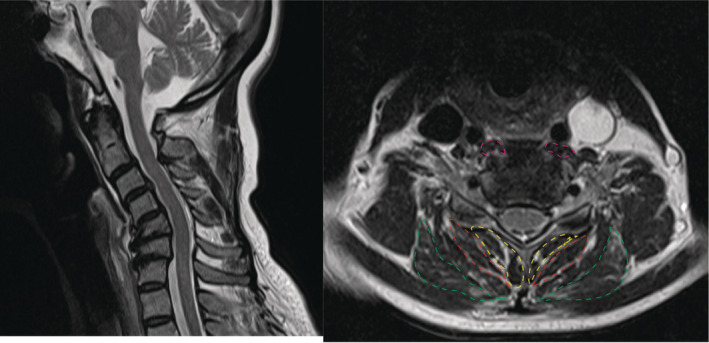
Magnetic resonance images depicting dropped head syndrome. Left and right images reveal the transverse relaxation time (T2)‐weighted sagittal, and axial images of the cervical spine (fifth/sixth cervical vertebral [C5/6] disc level), respectively. Magenta, red, green, and yellow, depict the longus colli, semispinalis cervicis, splenius capitis, and multifidus muscles, respectively.
